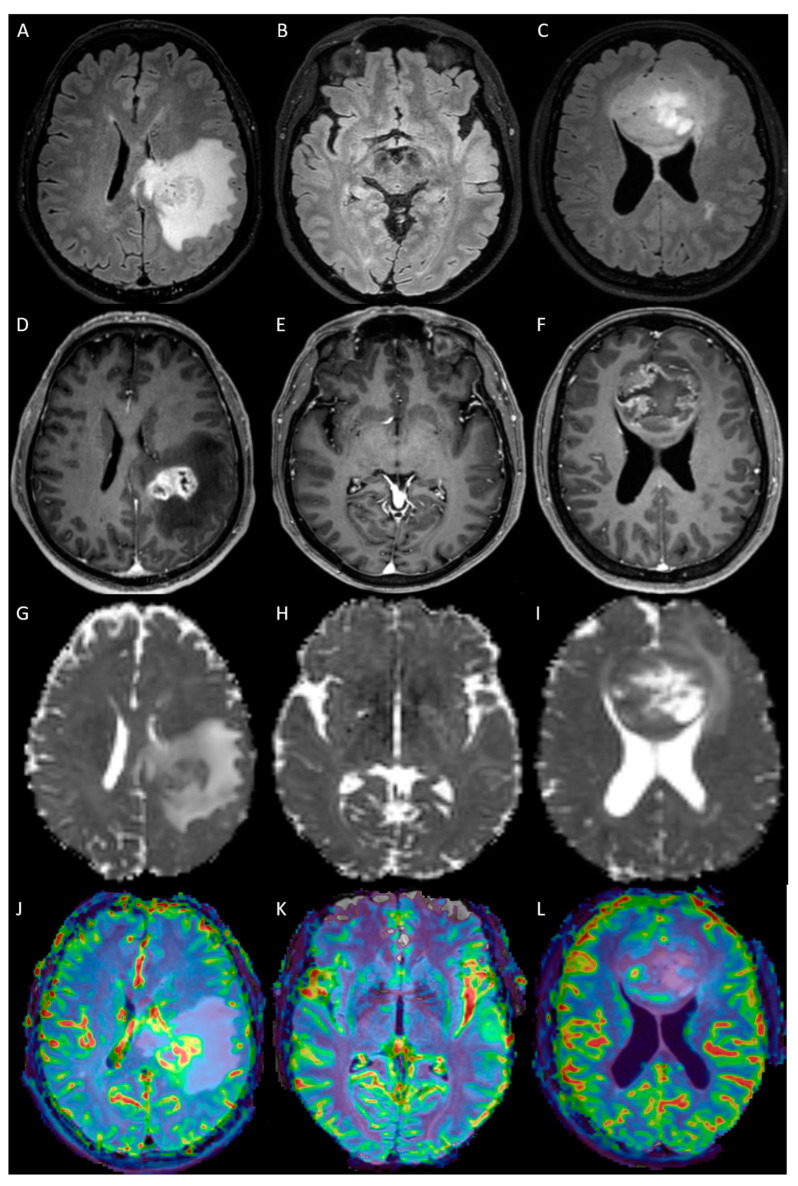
Preoperative MR images of three patients with WHO CNS grade 4 gliomas. The first column shows an example of an astrocytoma of WHO CNS grade 4 (*IDH* mutant). The second column shows images of a patient with only molecularly defined glioblastoma (*IDH* wild type). Note that there is no visible contrast enhancement in the contrast-enhanced T1-weighted image (D–F). In contrast, the third column depicts a case of “classical” glioblastoma showing typical irregular contrast enhancement surrounding a necrotic tumor core. The sequences depicted are T2-FLAIR (A–C), contrast-enhanced T1-weighted imaging (D–F), apparent diffusion coefficient maps (A,C,D,G–I), and leakage-corrected relative cerebral blood volume maps (rCBV, (J–L)).
